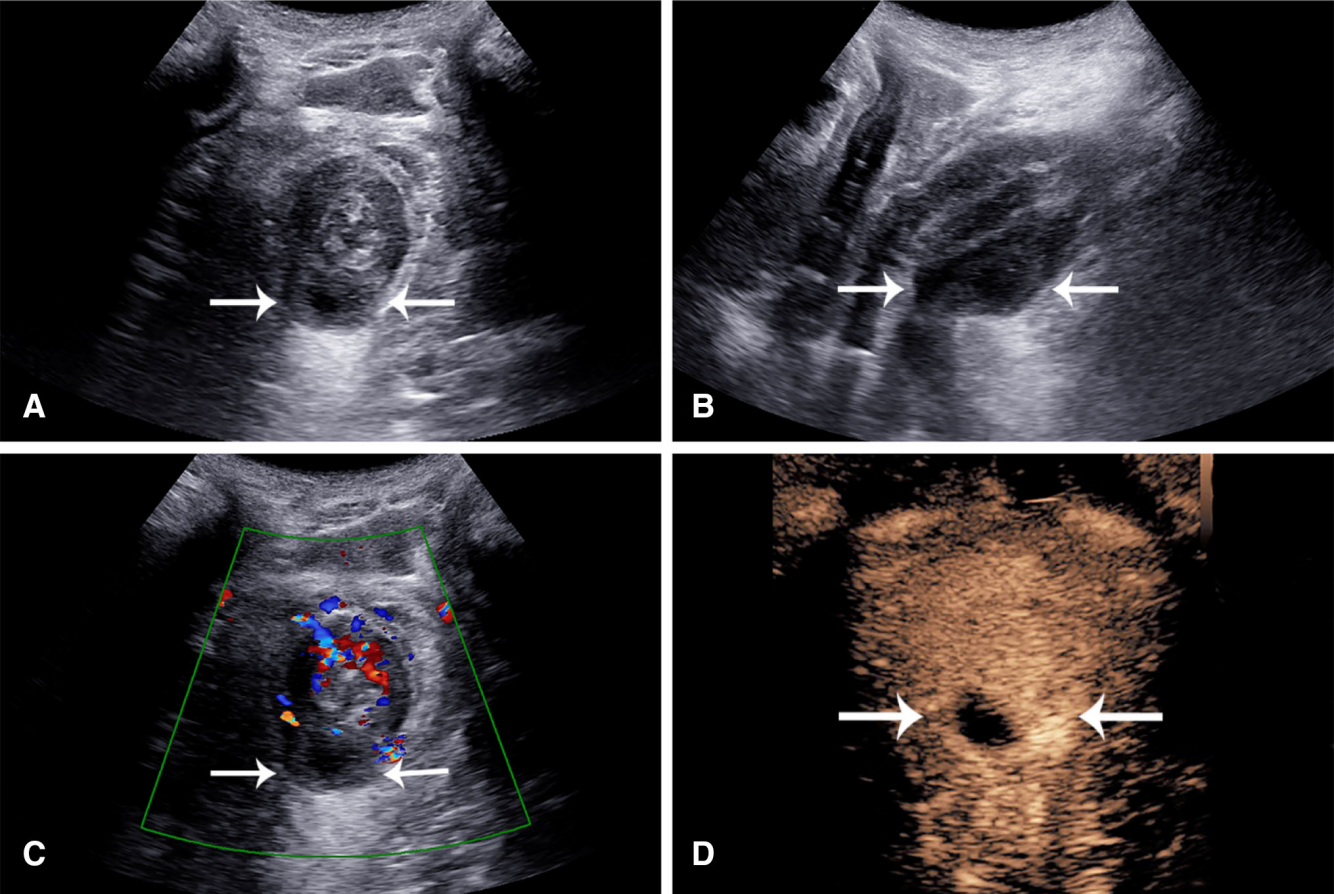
A 53-year-old male with CD for one year with very few symptoms. A Axial and B sagittal grayscale perianal scan shows a perianal intramural hypoechoic mass involving the posterior anal canal from 6 to 8 o’clock (arrows). C Color Doppler of the same region shows hypervascularity of the anal canal and no color signal within the mass. D CEUS shows peripheral enhancement of the mass with a central avascular component suggesting pus, consistent with an abscess cavity (Supplementary Video 4)
